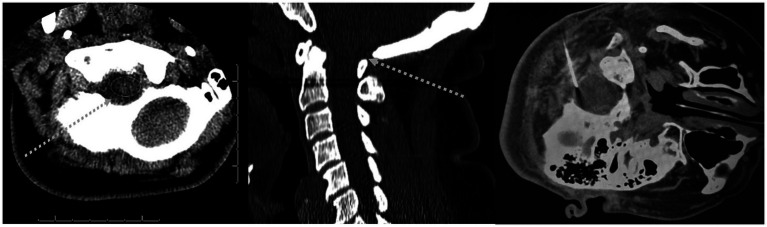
Suboccipital approach. (Left and middle panels): Dashed arrow indicates off-plane needle trajectory on a typical diagnostic CT. (Right panel): CT-guided sub-occipital needle placement.
